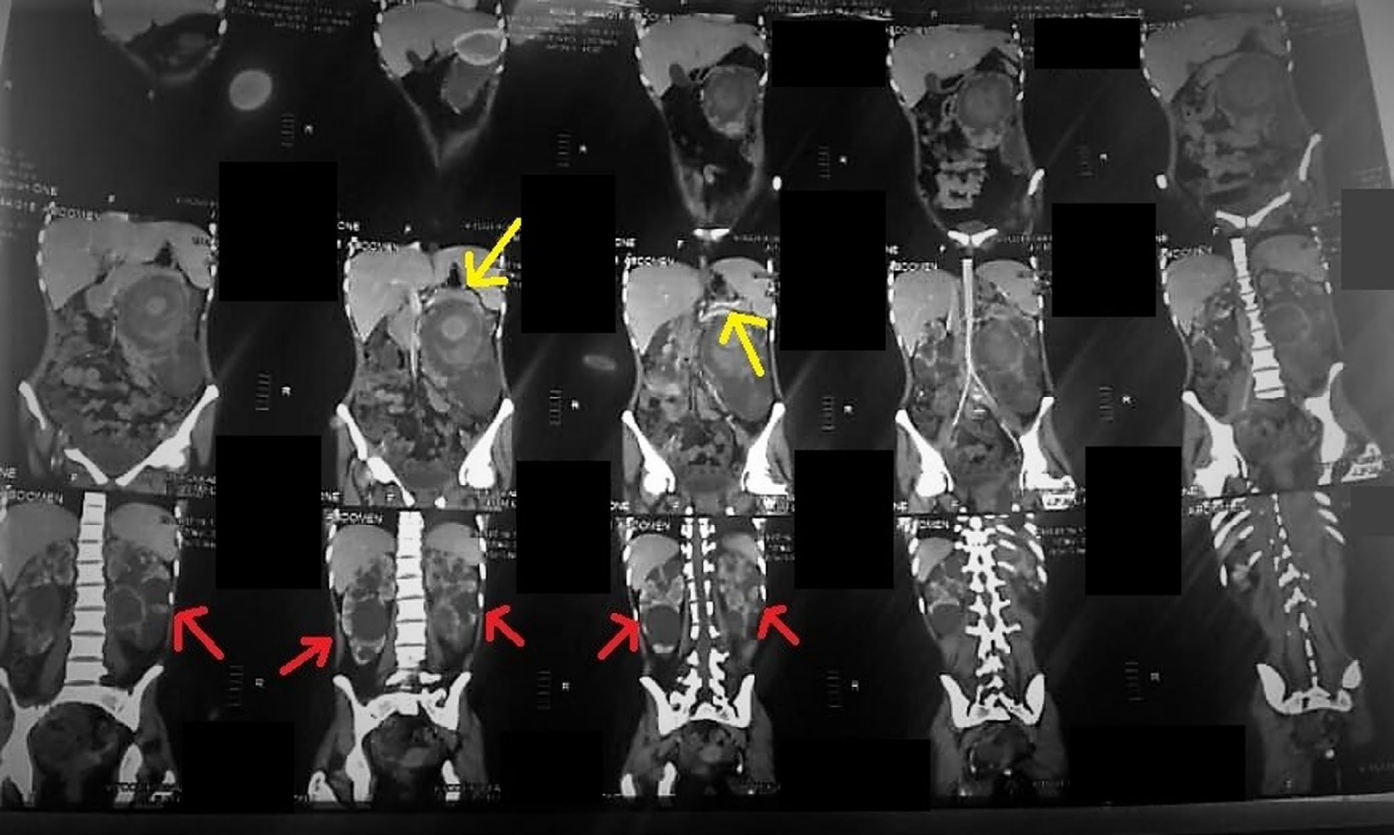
CT abdomen and pelvis shows swollen distal body and tail of pancreas (yellow), with few para‐aortic lymph nodes. Bilateral polycystic kidney disease (red) can also be seen
